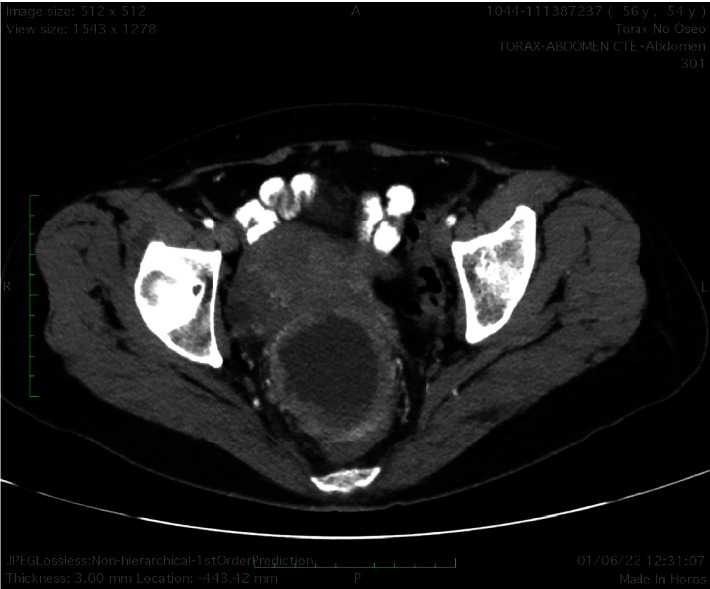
Contrast-enhanced computed tomography (CT) scan revealing a cystic tumor. Axial slice of a contrasted tomography of the abdomen visualized at the level of the pelvis where a circumscribed hypodense image is observed, and with a thick wall with enhancement to the contrast, as well as loss of the interface with the uterus and rectum, both are displaced.
